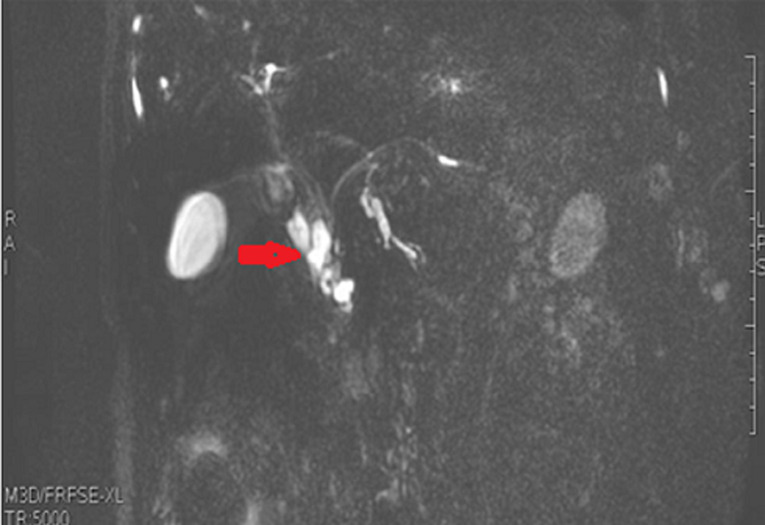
pancreatic magnetic resonance imaging showed branch duct intraductal papillary mucinous neoplasm of the head of the pancreas
